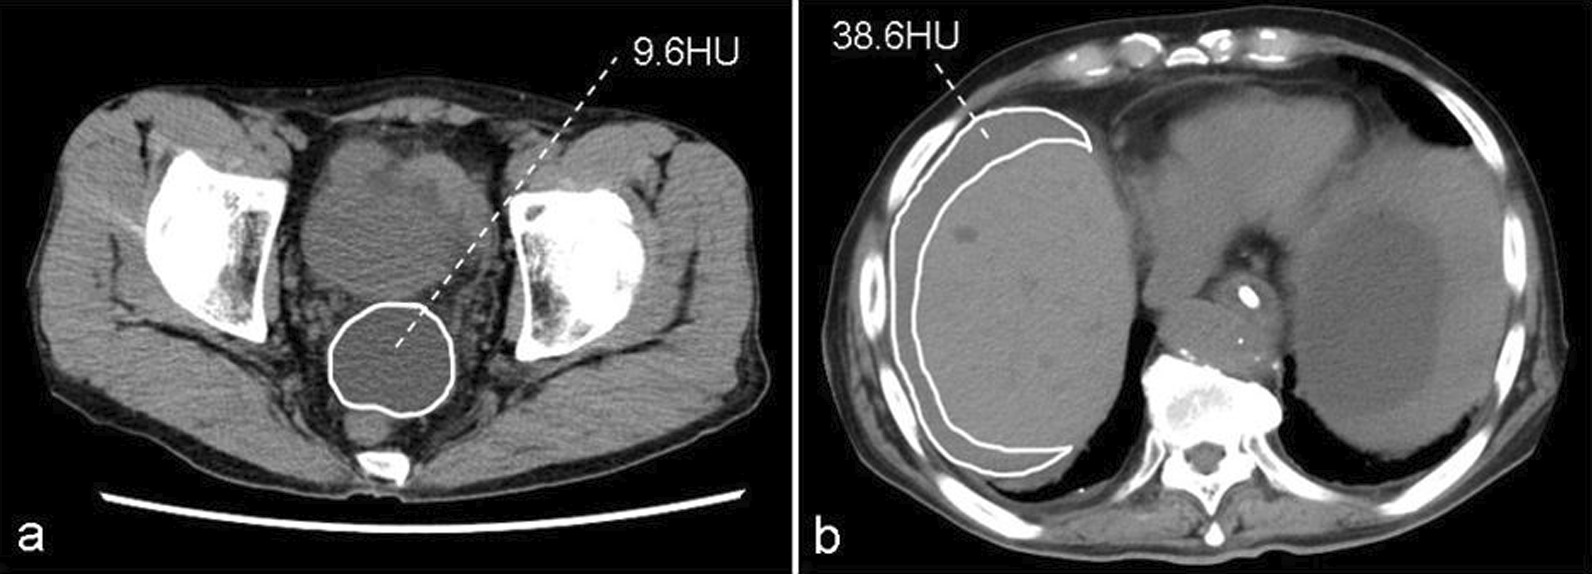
CT values of peritoneal fluid. Free peritoneal fluid in the rectovesical pouch (a) and below the right diaphragm (b) on non-enhanced computed tomography (CT) with mean CT values of 9.6 Hounsfield units (HU) and 38.6 HU, respectively, using a region-of-interest analysis
